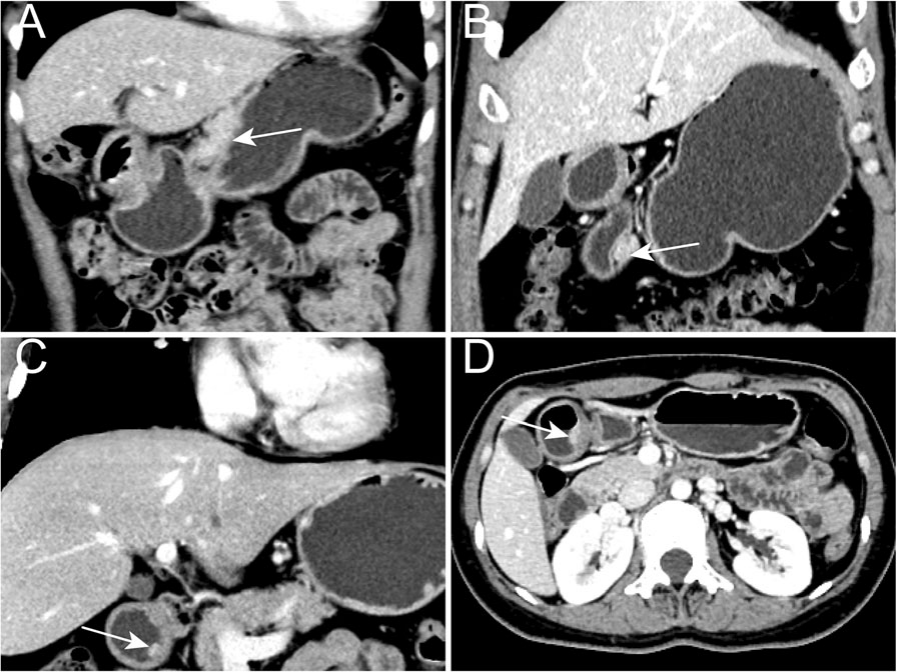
Representative CT images in the venous phase of heterotopic pancreatic masses (white arrows). a The coronal image shows an ill-defined irregular mass in the gastric middle body with exo-luminal growth pattern. b The coronal image shows an ill-defined ovoid mass in the gastric lower body with mixed growth pattern. c The coronal image shows a will-defined flat mass in the gastric lower body with endo-luminal growth pattern. d The axial image shows an ill-defined hill-like mass in the gastric lower body with endo-luminal growth pattern. The LD/SD ratio of this lesion is 1.85 (15.83/8.54 mm). The relative enhancement ratio of HP masses to the pancreas is 0.94 (101.25/107.58 HU)
